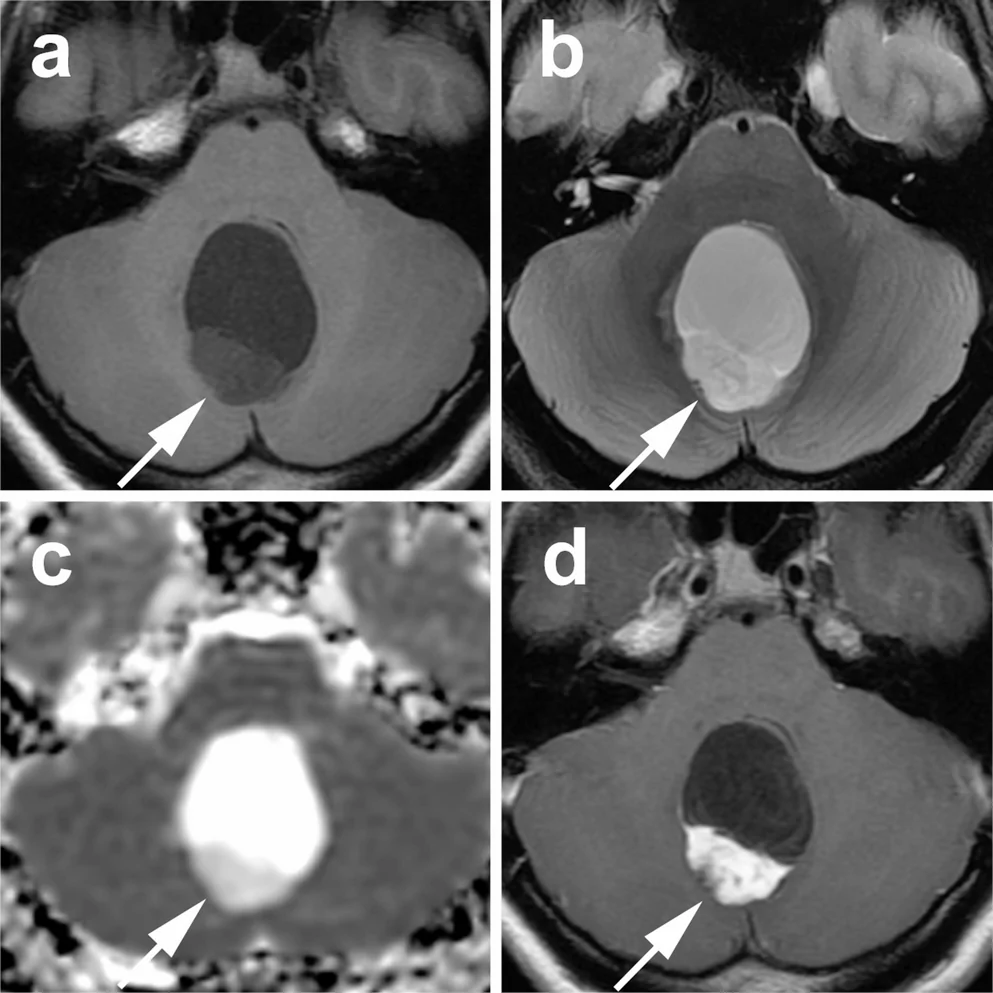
A 19-year-old woman with pilocytic astrocytoma presenting with dizziness, gait disturbance, headache, and vomiting. Axial MR images demonstrate a cystic component with a dorsally located solid portion (arrows) that exhibits low signal intensity on T1-weighted images (a), high signal intensity on T2-weighted images (b), high ADC values (c), and contrast enhancement (d). The fourth ventricle is compressed by the tumor
